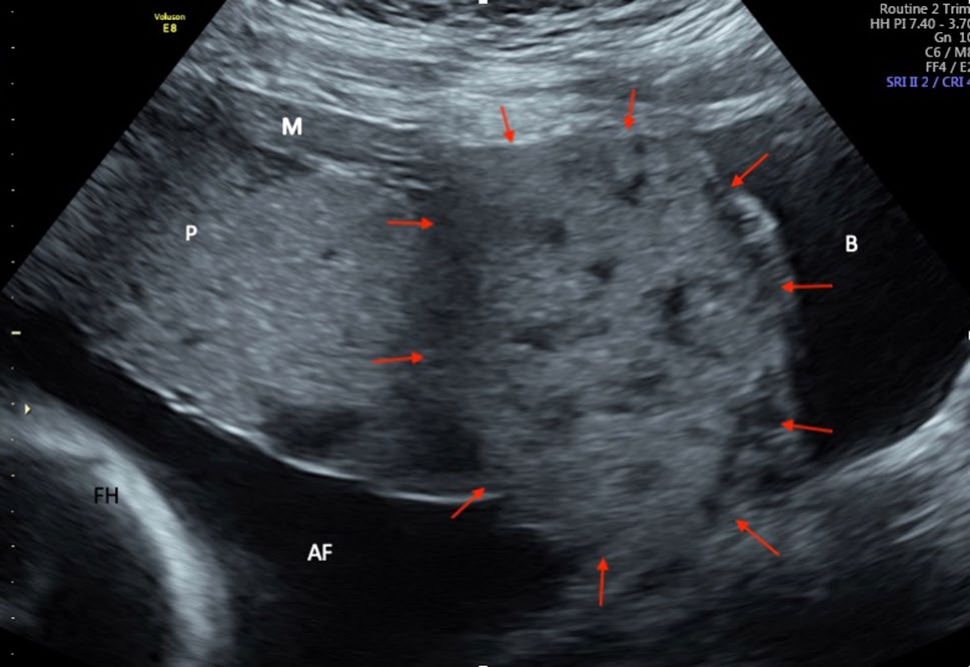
Abdominal sonography in 22 + 6 weeks of gestation, sagittal view, b-mode. Suspected percrete area of the placenta (marked with arrows) with typical bulging into the bladder (B) and thinning of the myometrium (M). Fetal head (FH), placenta (P), amniotic fluid (AF)
